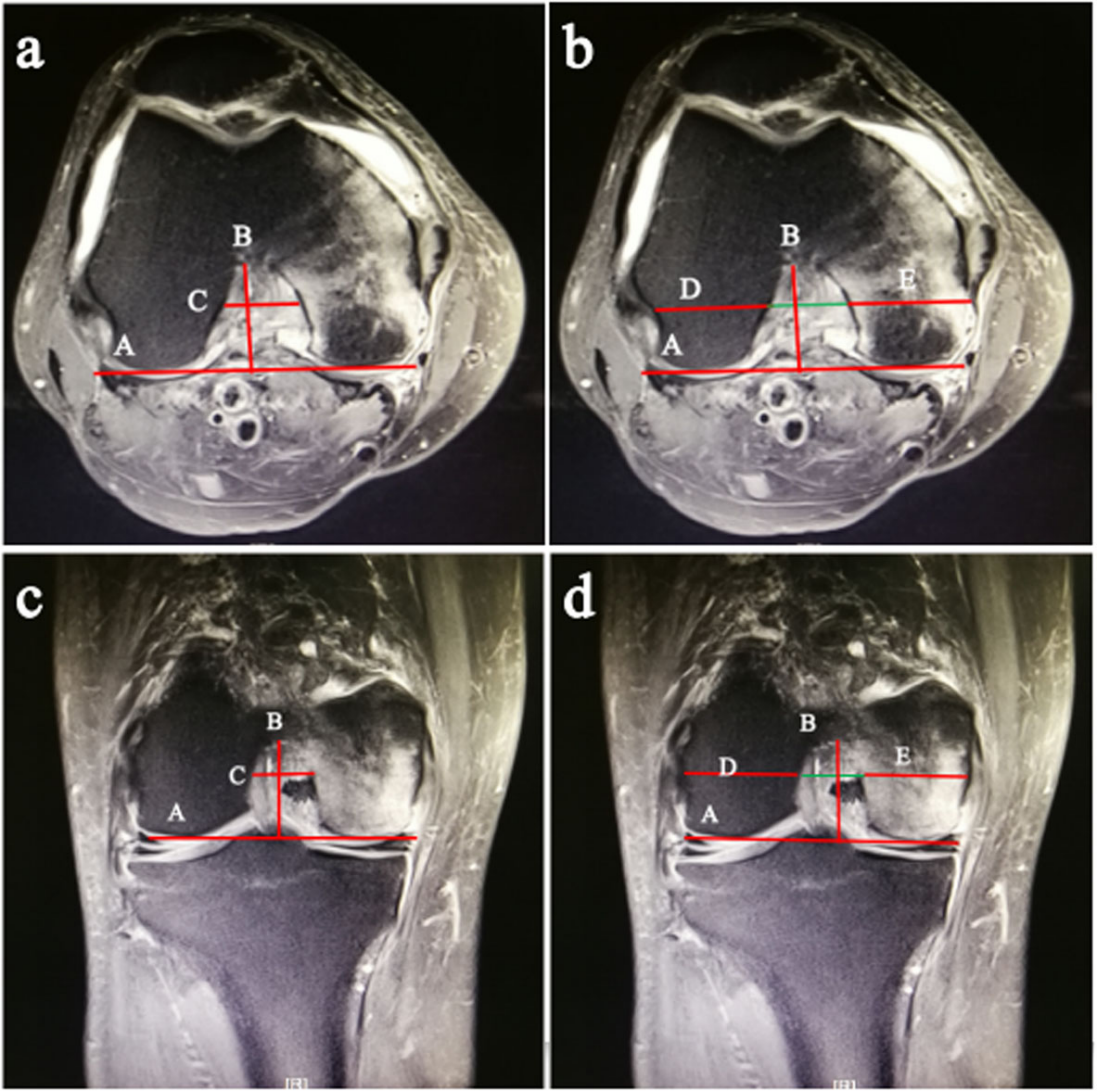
Magnetic resonance images depicting the method used to obtain the femoral measurements in the axial plane. a The intercondylar height (line B) was the distance from the top of the intercondylar notch to the bicondylar line (line A). The intercondylar width (line C) was obtained at the anterior third of the intercondylar height (line B). b At the same level, the width of the lateral and medial condyles (line D, E) was obtained. The same method was used to collect these measurements in the coronal plane (c and d)
